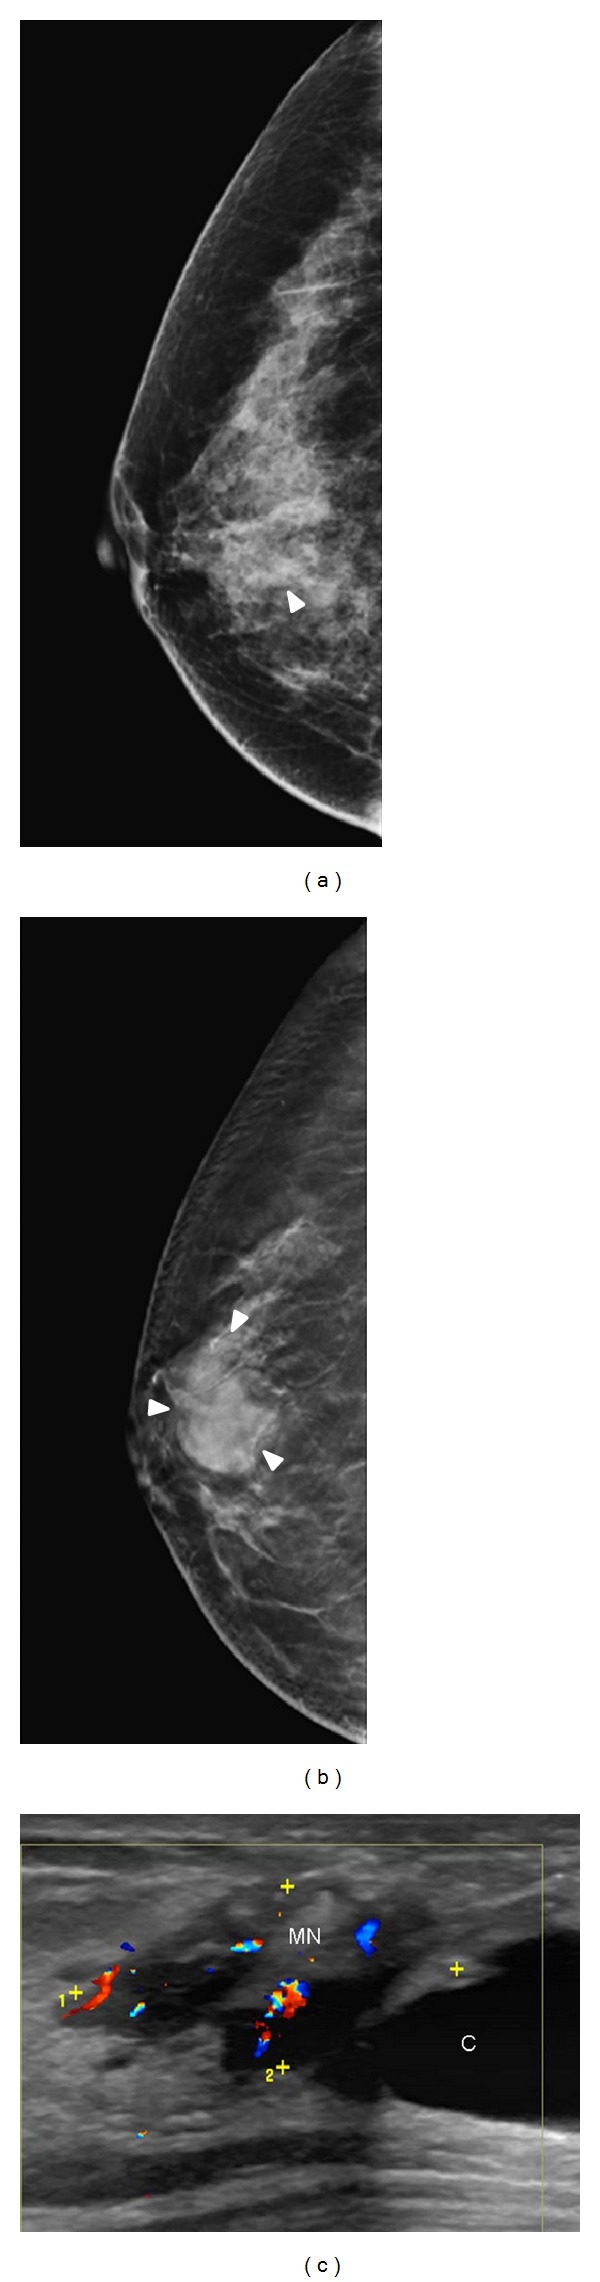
A 61-year-old woman with palpable mass in the right breast. (a) Digital mammograms showed focal increased density (arrowhead) without architectural distortion in the right breast. (b) Tomosynthesis clearly delineated the smooth border of a mass lesion (arrowhead) without showing malignant feature. This case was considered as true negative in both digital mammography and tomosynthesis. (c) Color ultrasound showed a cystic-like lesion (C) with hypervascular mural nodule (MN). The lesion was proved to be ductal carcinoma in situ, TisN0M0.
